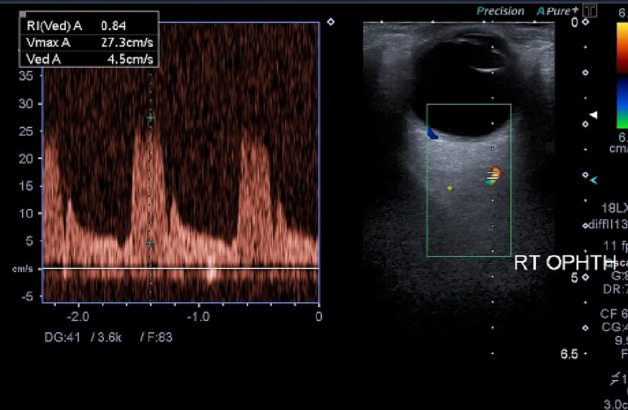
CDI of the right eye of a diabetic patient showing the Doppler spectral waveform of OA at the intersection of the OA with the Optic Nerve. Note the characteristic waveform of the OA showing the high-resistance flow with high systole and low diastole.
